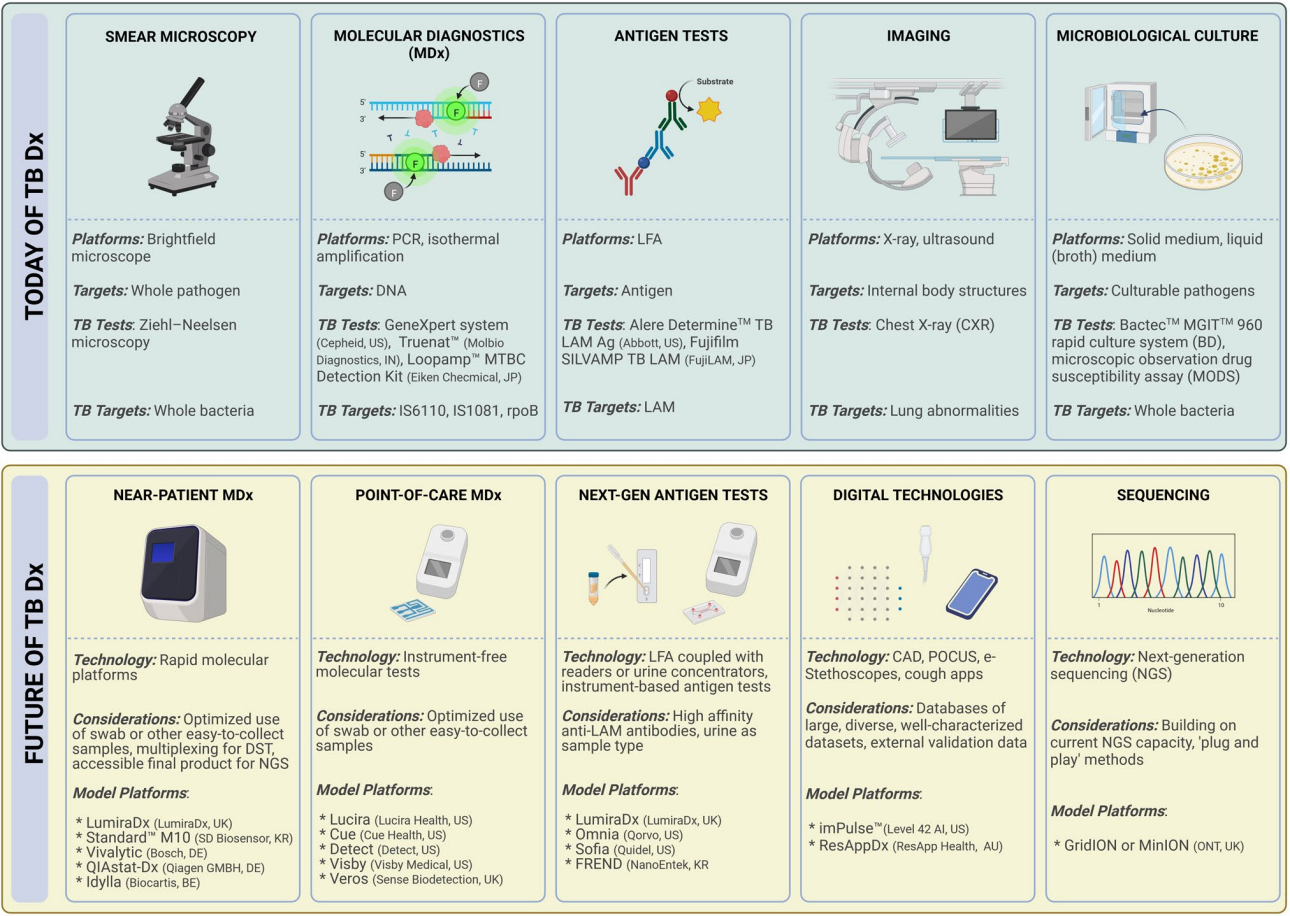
Today and future of TB diagnostics (Dx). IS6110: insertion sequence 6110; IS1081: insertion sequence 1081; *DST* drug-susceptibility testing, *NGS* next-generation sequencing, *LFA* lateral flow assay, *LAM* lipoarabinomannan, *CAD* computer-aided detection, *POCUS* point-of-care ultrasound. Figure created with BioRender.com
